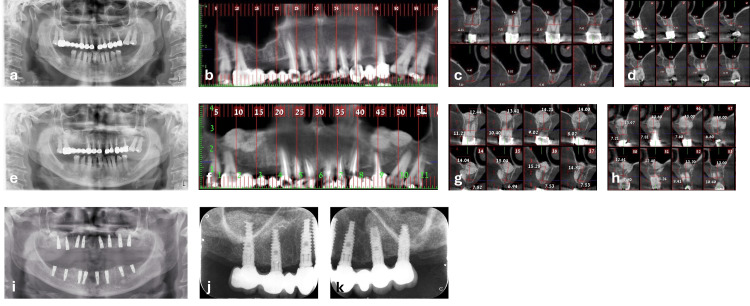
Preoperative and postoperative radiographic views of case 2. (a) Preoperative panoramic radiograph showing hopeless posterior maxillary teeth bilaterally and vertical bone deficiency. (b-d) Preoperative cone-beam computed tomography (CBCT) images confirming the vertical bone deficiency at the posterior maxilla. (e) Postoperative panoramic radiograph following bilateral sinus augmentation, performed prior to tooth extraction. (f-h) Postoperative CBCT images demonstrating new bone formation following bilateral sinus lift procedures. (i) Panoramic radiograph after the second surgical stage, including tooth extractions and implant placement. (j-k) Follow-up radiographs at 10.5 years postoperatively.
